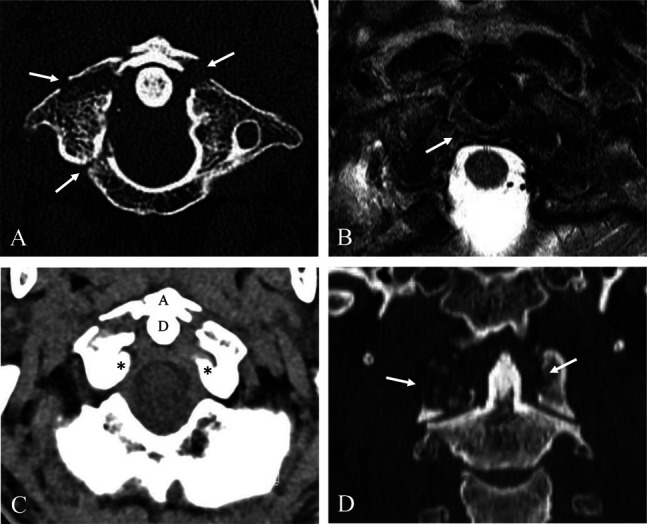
Axial CT scans (A and C) showing a three-part Jefferson fracture (white arrows) and a posteriorly displaced occiput (arrows) about the anterior arch of the atlas and the dens of the axis. Coronal reconstructed CT scan (D) reveals fractures of the atlas (white arrows). Axial MRI (B) shows the rupture of the transverse atlantal ligament at C1-2 (white arrow).
